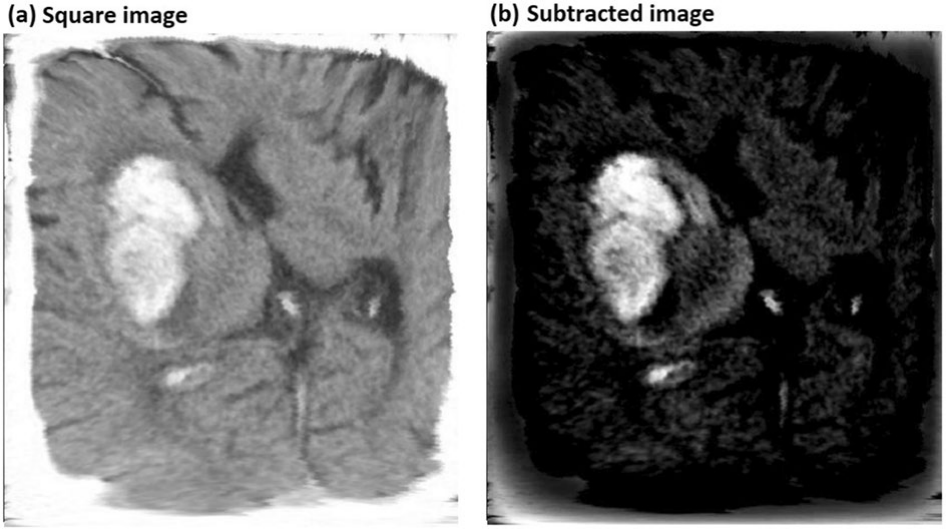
Example of CT image processing for preparing image data. (a) Square image expanded to fit in a square. (b) Subtracted image to eliminate the skull image and other images not related to ICH.
